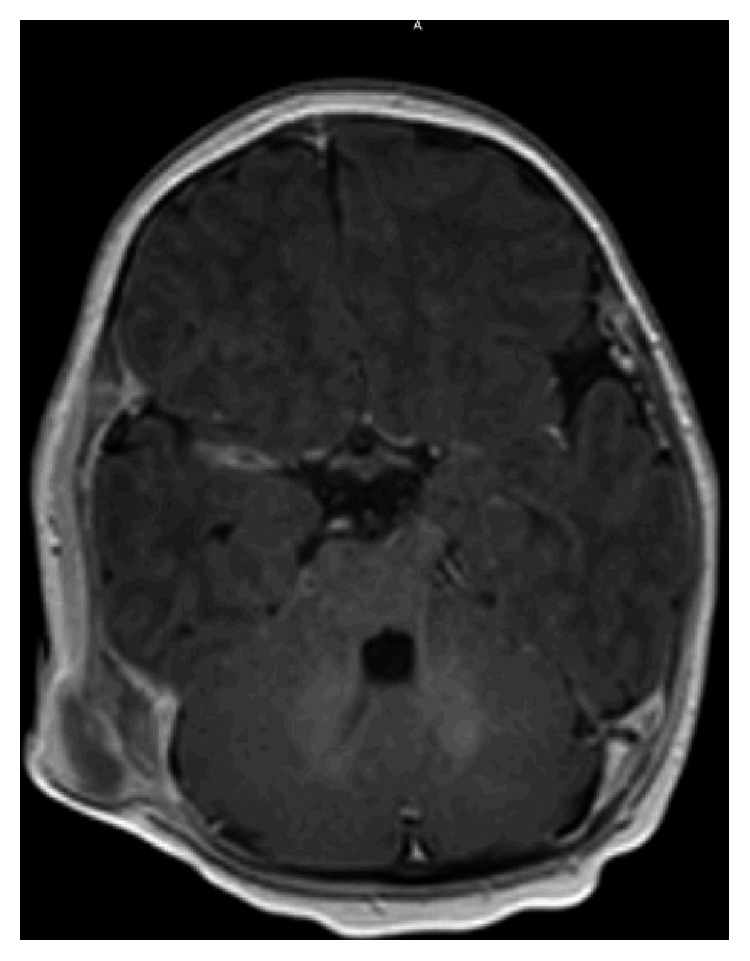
T1-contrasted sequence MRI of the initial lesion prior to any surgical therapy. Note: isointensity with signal abnormality of the adjacent temporal bone and temporoparietal suture line and peripheral enhancement representing areas of nonossification.
